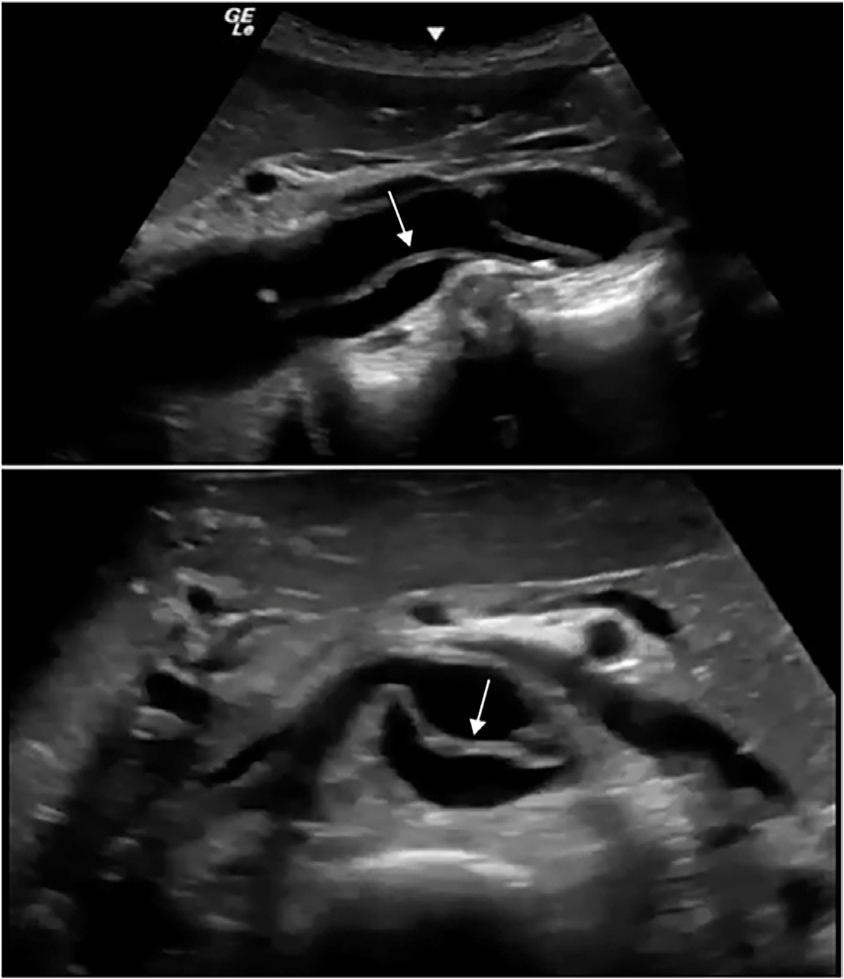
Sagittal (top) and transverse (bottom) ultrasonography view of abdominal aorta. Two views of the abdominal aorta. An arrow points to a large, undulating intimal flap noted within the abdominal aorta.
